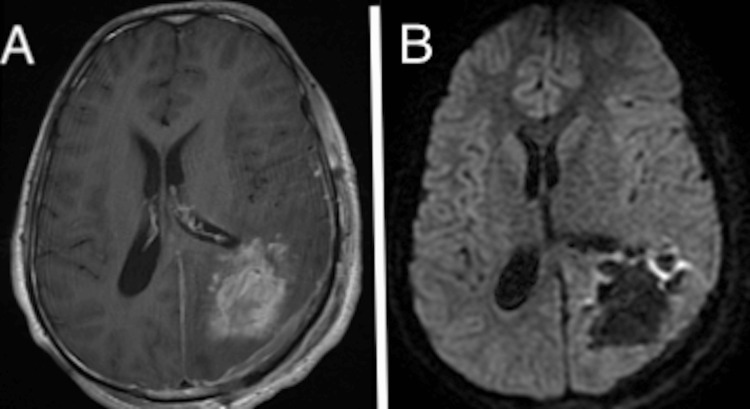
Postoperative brain MRI showing total resection with the disappearance of the mass effect. (A) Axial T1 postcontrast and (B) DWI. DWI: Diffusion-weighted imaging.
